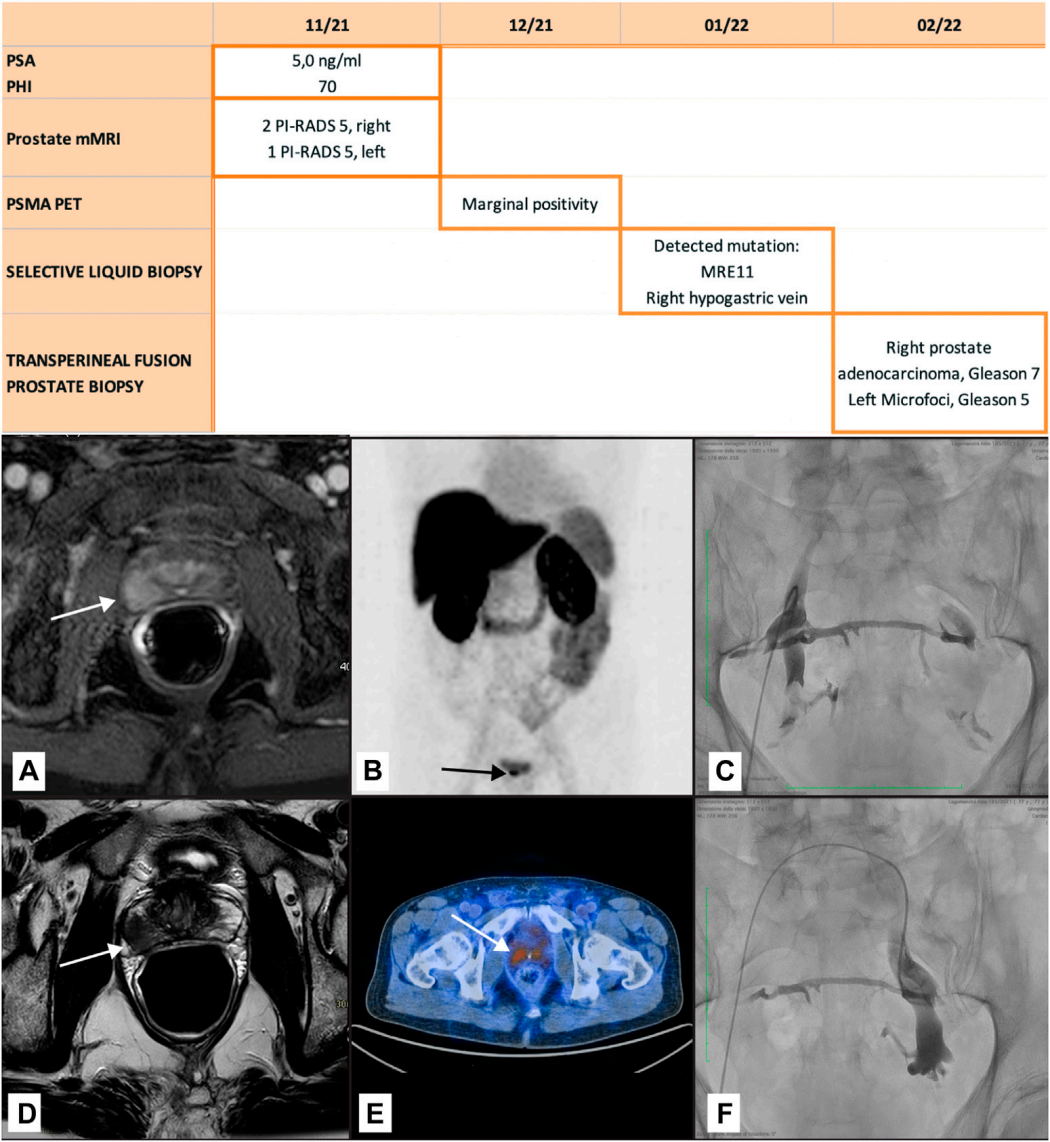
Prostate adenocarcinoma. The table shows the tests perfomed. The figures show the multiparametric MRI detecting the tumor (B, C), the PSMA with marginal positivity (D), and right transfemoral catheterization of the hypogastric veins for ctDNA sampling (E, F) with the veins opacified by injection of contrast medium. The two sides are connected by the pelvic venous plexus.
